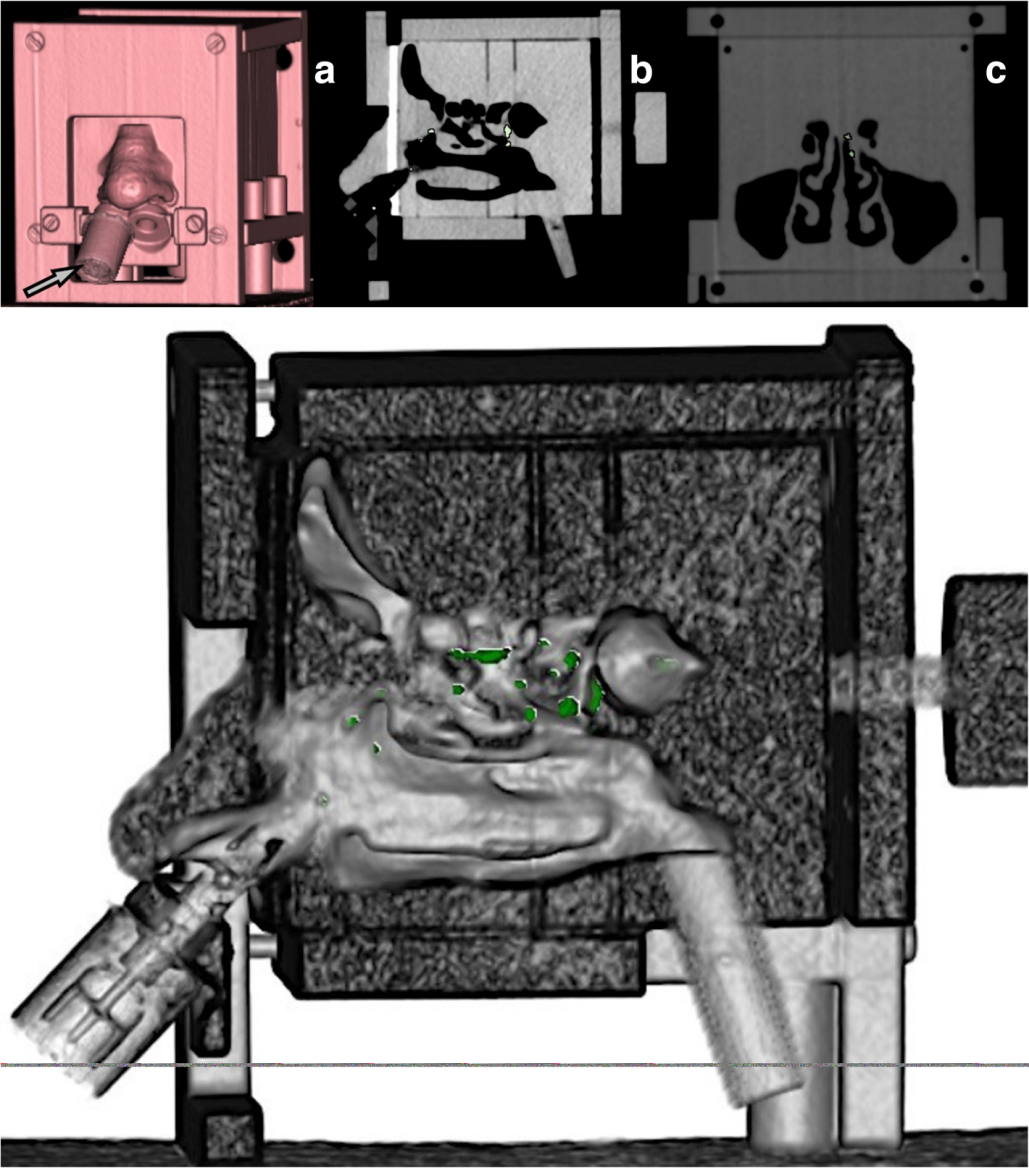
Three-dimensional volume-rendered computed tomography image demonstrating the nasal cast from anteriorly: the nose spray (arrow) was applied into the support below the right nostril for standardised application of the spray (a). Sagittal reformation depicting the iodinated contrast agent droplets in the nasal cavity (bright hyperdense spots) (b). The colours indicate pixels with attenuation values exceeding the predefined threshold in the region of interest (c). On the lower image, a sagittal image after segmentation is shown. The green colour indicates the iodinated deposited droplets. Note the depositions mainly in regions next to the superior and middle concha
